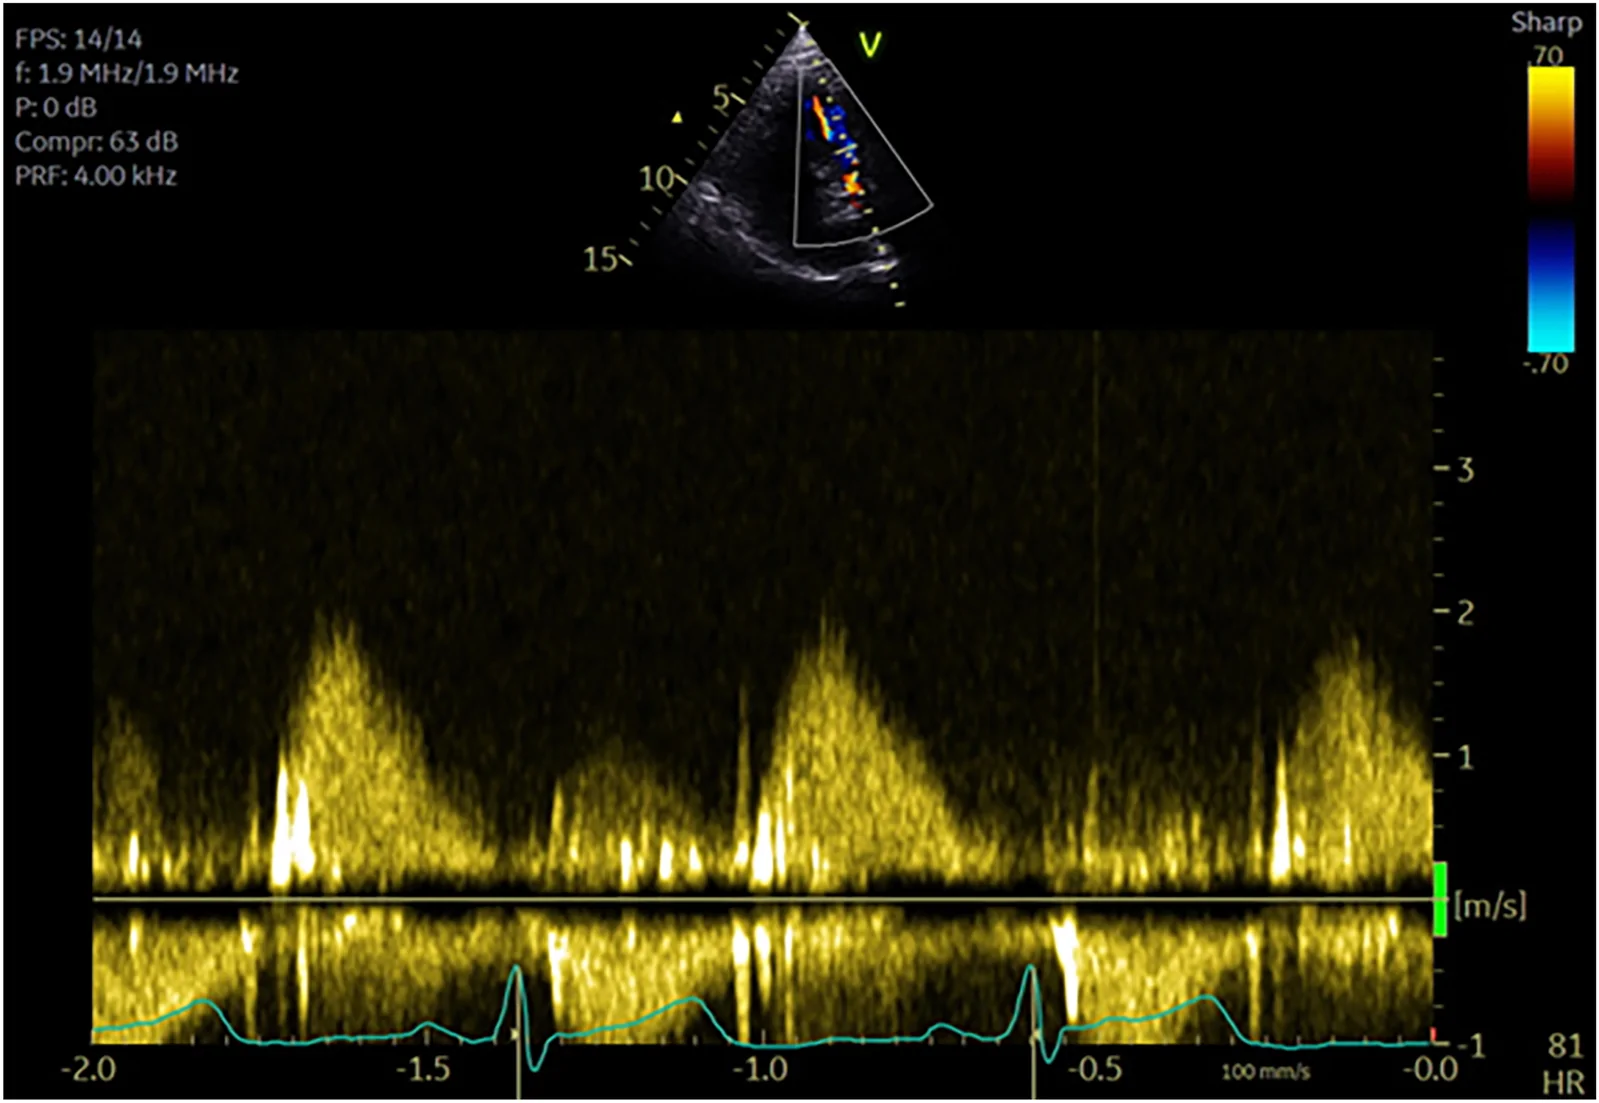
Continuous-wave spectral Doppler display with the cursor across the PV demonstrates a dense, diastolic spectral Doppler profile with rapid deceleration and early termination of flow, consistent with severe PR.
